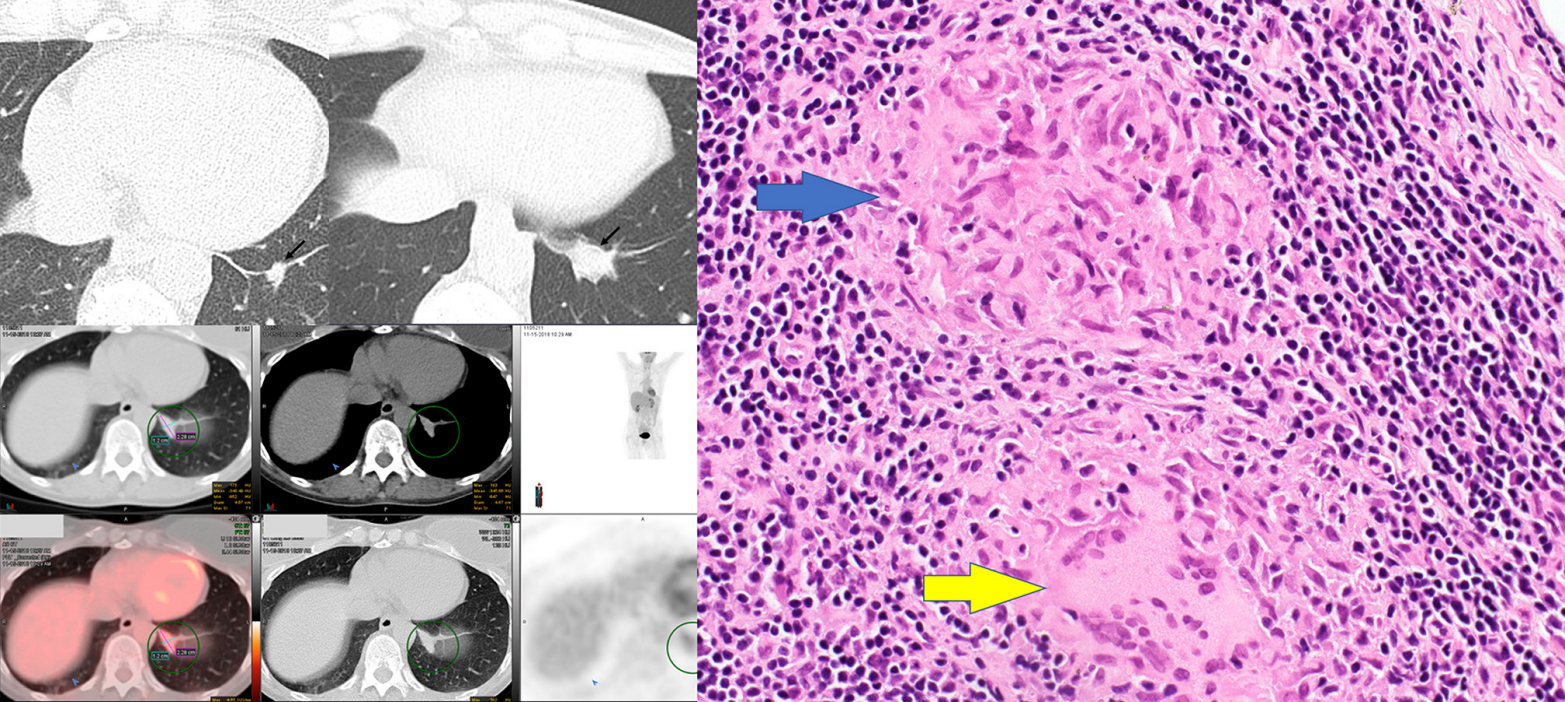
A–D. 57-year-old asymptomatic female with path-proven granulomatous lymphadenitis. 2A: Axial coronary chest CT image on lung windows showing an incidental 14 mm lobulated solid nodule in the left IPL. 2B: Axial chest CT image on lung windows obtained 6 months later demonstrating significant enlargement of the nodule to 2.2 cm. 2C: PET CT of the lesion demonstrating FDG-avidity with SUV of 4.7, interpreted as “concerning for malignancy”. 2D: PATH slide following wedge resection of the lesion. The lymph node contains multiple aggregates of epithelioid macrophages with bent elongated nuclei (blue arrow) and aggregates of multinucleated giant cells (yellow arrow). FDG, fludeoxyglucose; PET, positron emmision tomographyPL, inferior pulmonary ligament; PET, positron emmision tomography.
